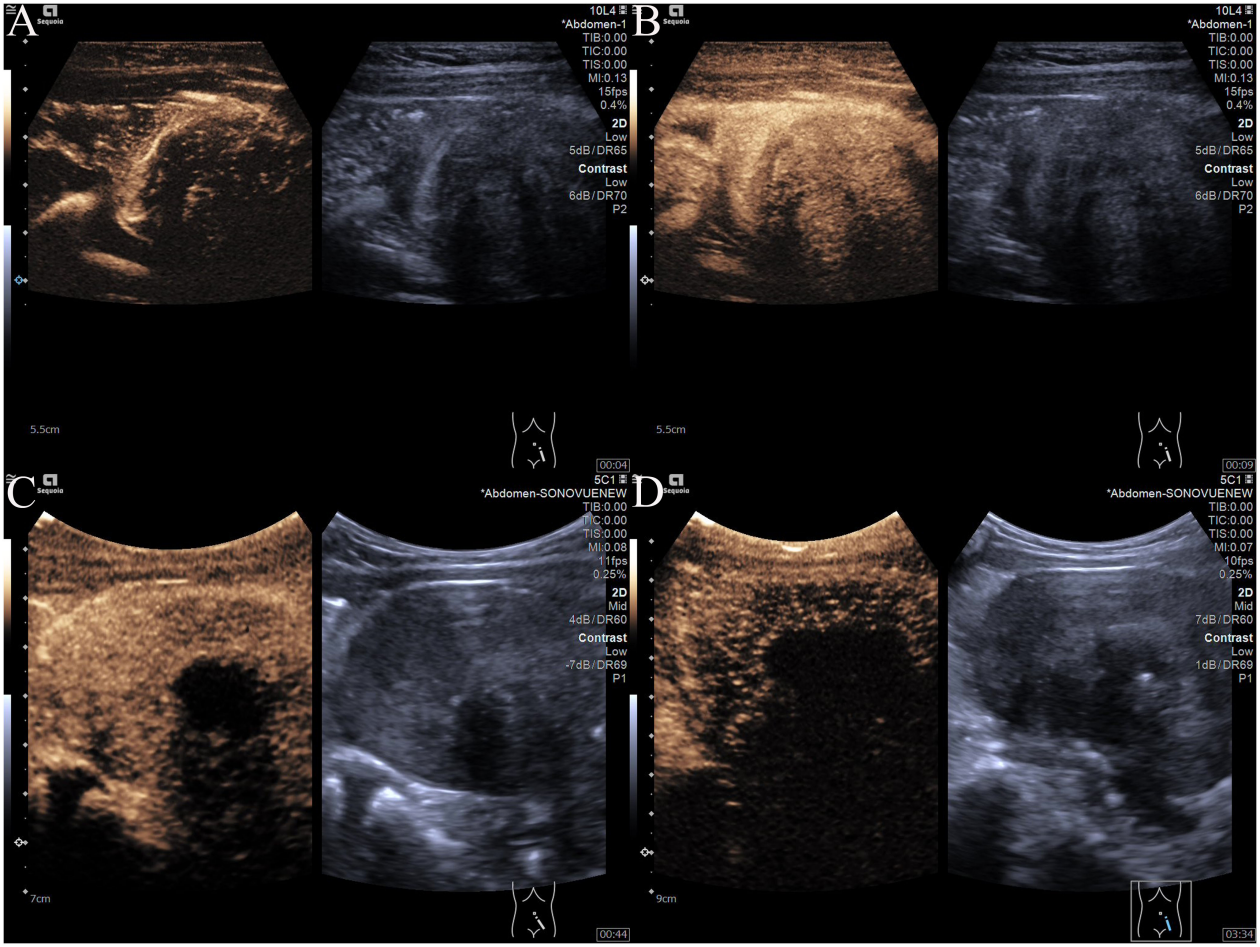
Contrast-enhanced ultrasound. (A) Arterial phase at 4 seconds: peripheral enhancement observed. (B) Peak enhancement at 9 seconds: slight hypo-enhancement detected. (C) Venous phase: hypo-enhancement with a central non-perfused area. (D) Enhancement persisting up to 3.5 minutes.
